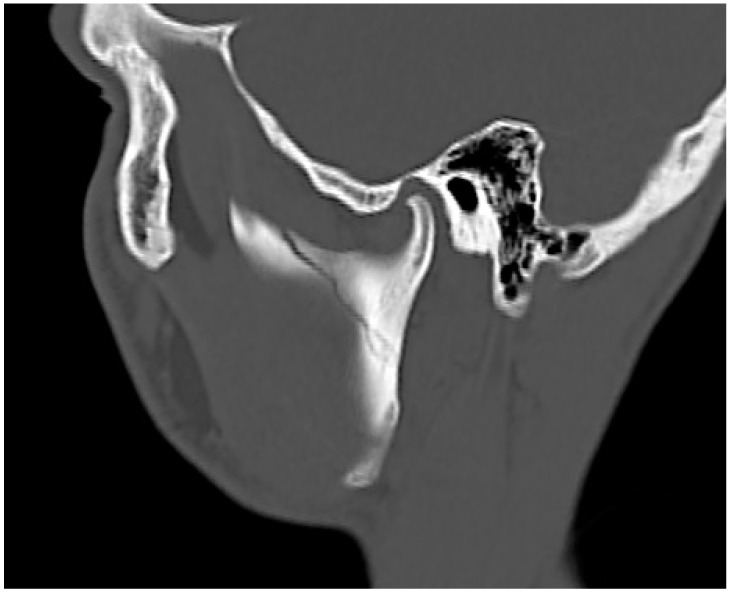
Computed tomography showing the presence of a linear fracture gap at the base of the left mandibular condyle without splinter displacement.
